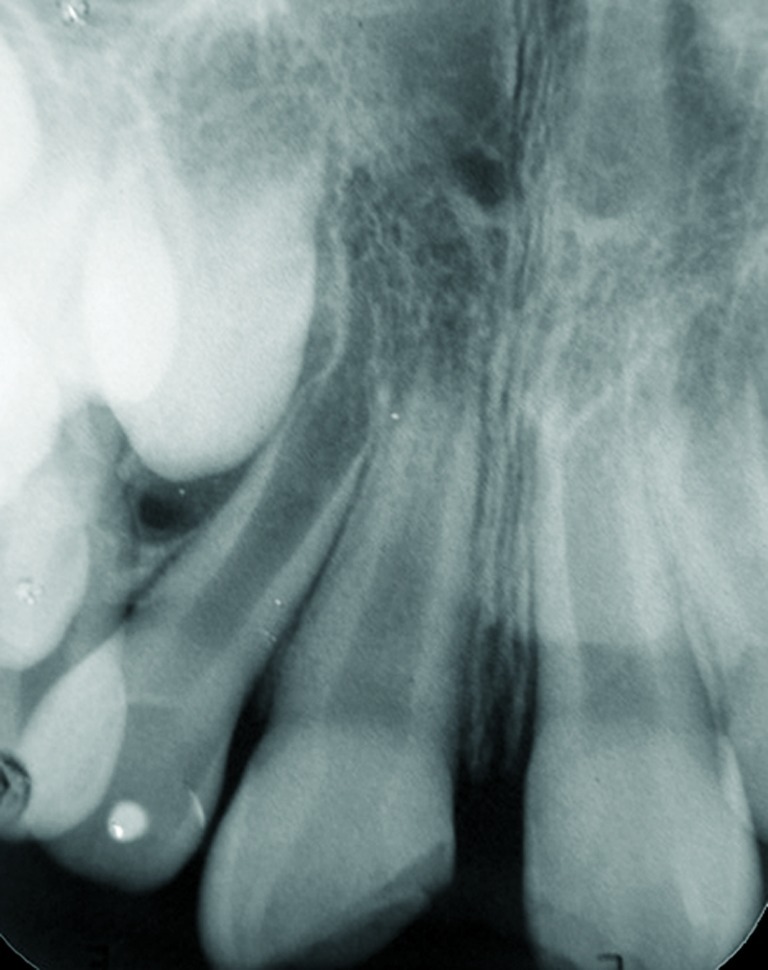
Initial periapical radiograph revealing incisors’ incomplete root formation
